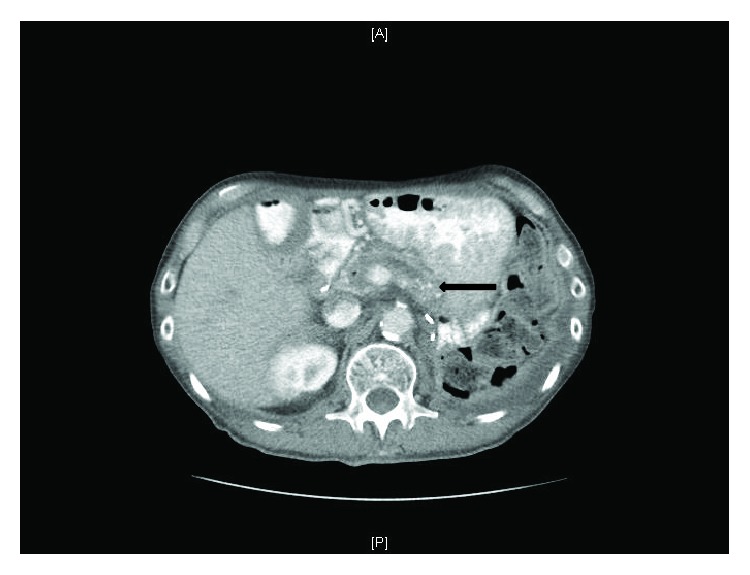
CT imaging. Evidence of severe chronic pancreatitis with calcifications (arrow) and dilation of the pancreatic duct.
